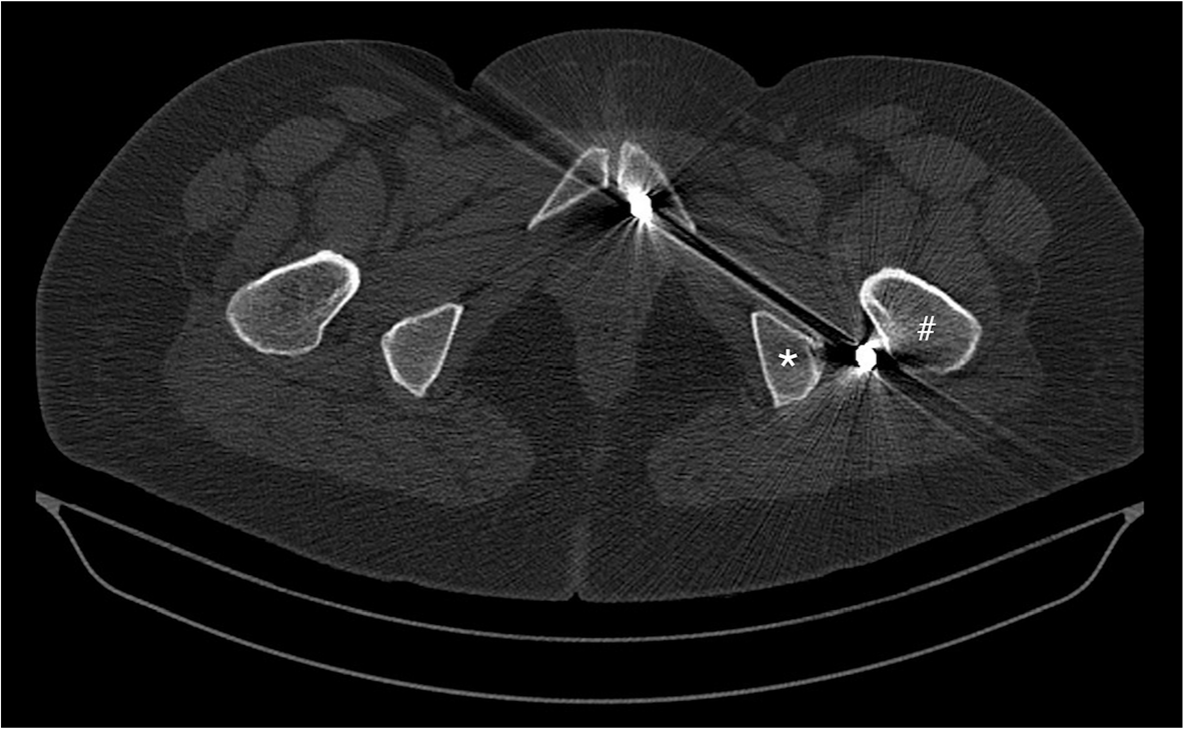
Preoperative computed tomography scan demonstrating the pellet of interest located between the trochanter major (#) and ischial tuberosity (*) close to the course of sciatic nerve
